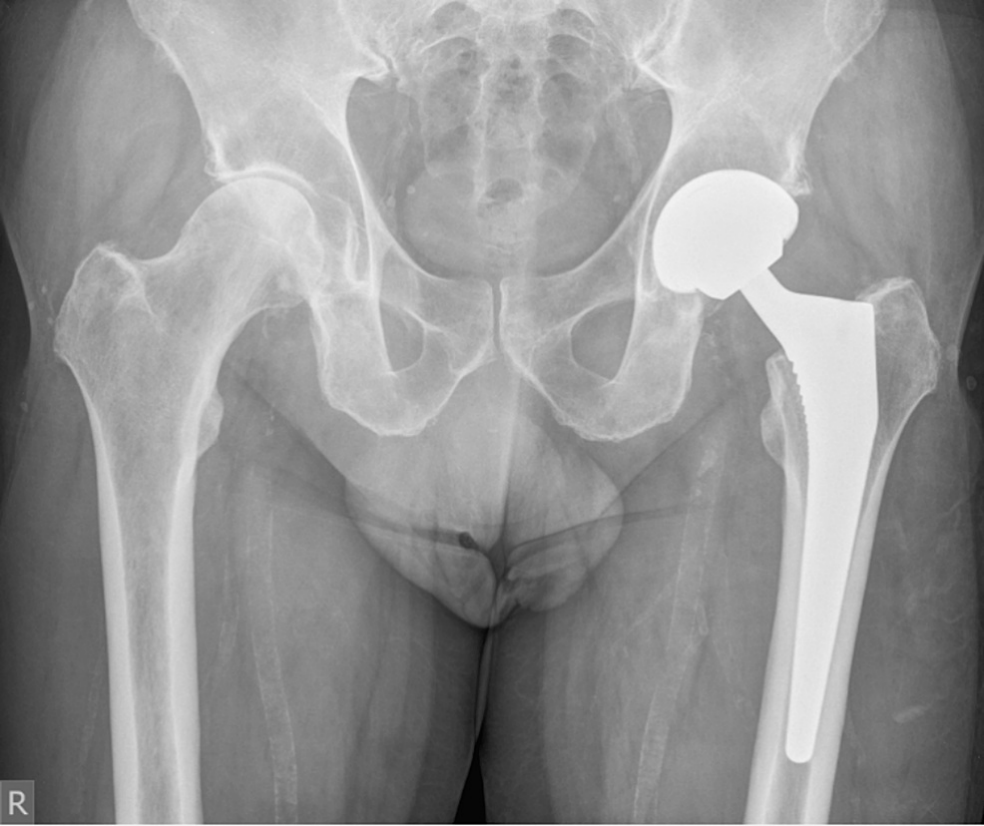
Standing anteroposterior radiograph showing a left high offset collarless Corail stem
